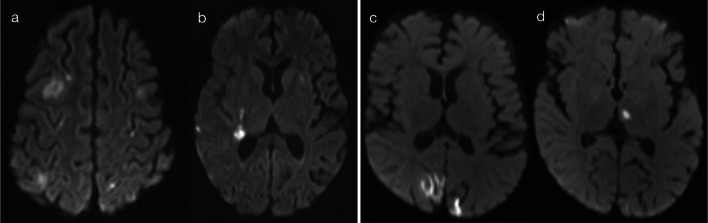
Restricted diffusion in supratentorial grey matter—scattered lesions (Pattern 2A). Case 1: 4-year-old child with somnolence and hyperaesthesia, in the setting of acute lymphoblastic leukaemia (ALL) and *Scedosporium prolificans* fungaemia. DWI (a, b) demonstrated scattered foci of restricted diffusion, mainly affecting cortical grey matter with a bilateral though asymmetric distribution. Case 2: 4-month-old infant with respiratory distress, in the setting of HIV, *Pneumocystis jiroveci* pneumonia and an abnormal cranial ultrasound. DWI (c, d) demonstrated scattered foci of restricted diffusion, mainly affecting cortical grey matter with a bilateral though asymmetric distribution
